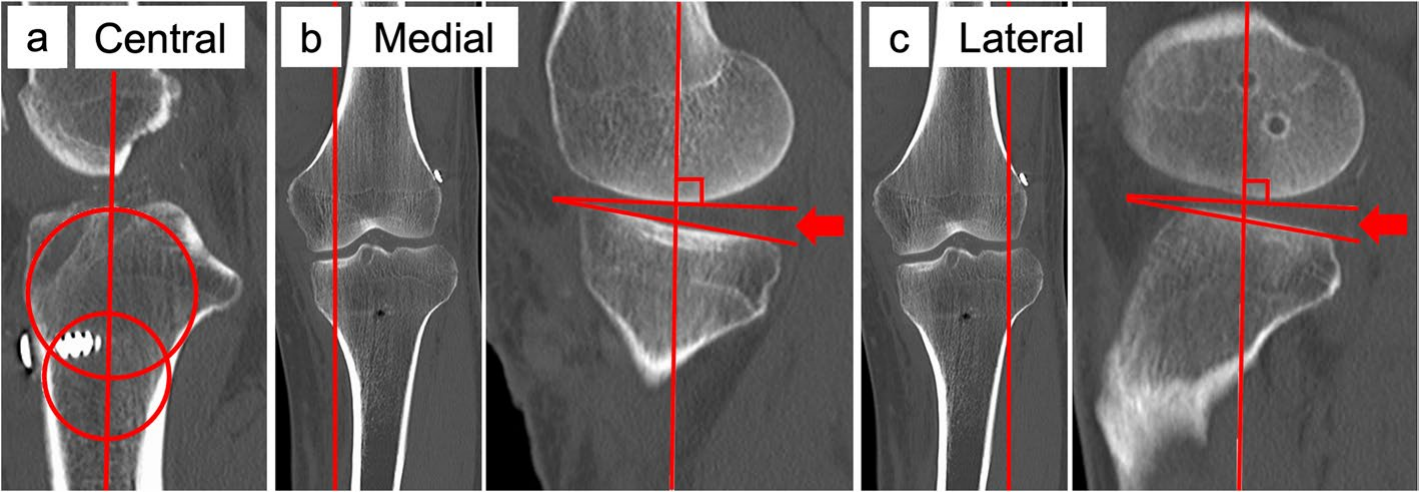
Posterior tibial slope (PTS) measurements using CT images. The arrow indicates the angle of lateral and medial PTS
